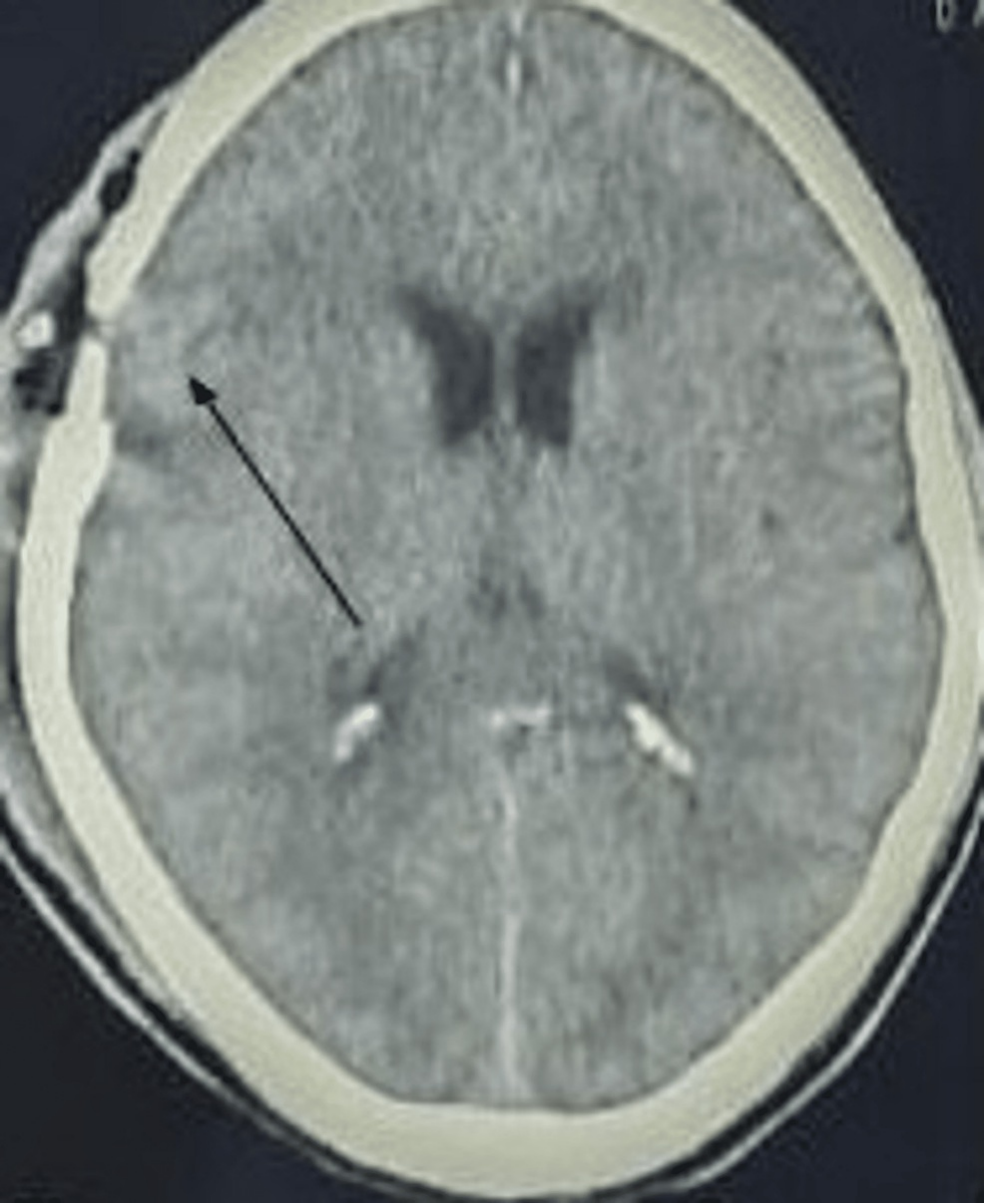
A post-operative CT scan of the brain with axial view showing evacuation of the hematoma after burr hole trephination of the chronic subdural hematoma (arrow)
